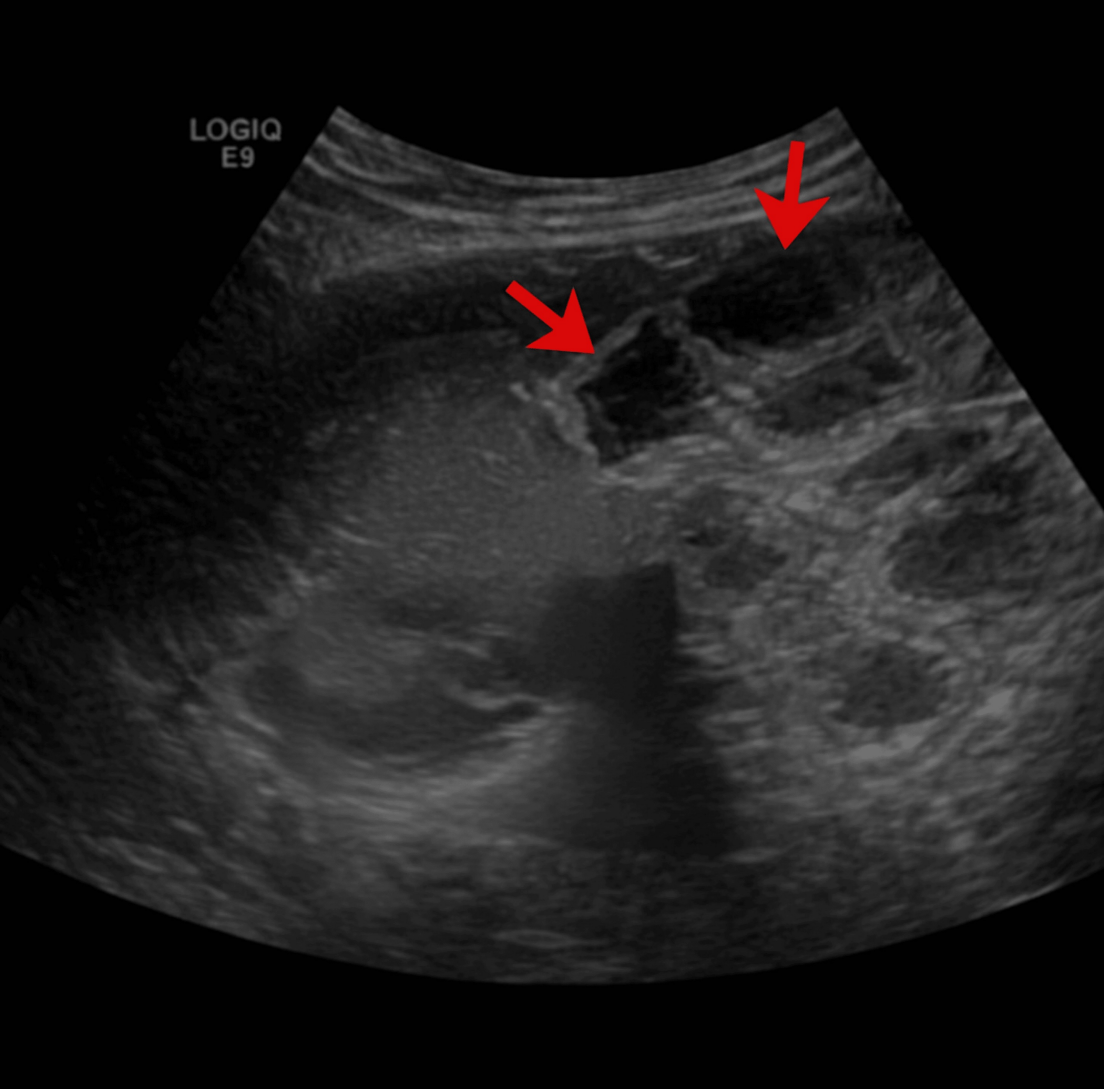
Ultrasound Abdomen This ultrasound image depicts severe hydronephrosis, characterized by the significant dilation of the renal pelvis and calyces (as indicated by the arrows), which appear as dark, fluid-filled (anechoic) pockets. The accumulation of trapped urine has distorted the kidney's normal architecture, creating a multiloculated appearance where the functional renal tissue, or parenchyma, appears thinned and compressed against the periphery.
